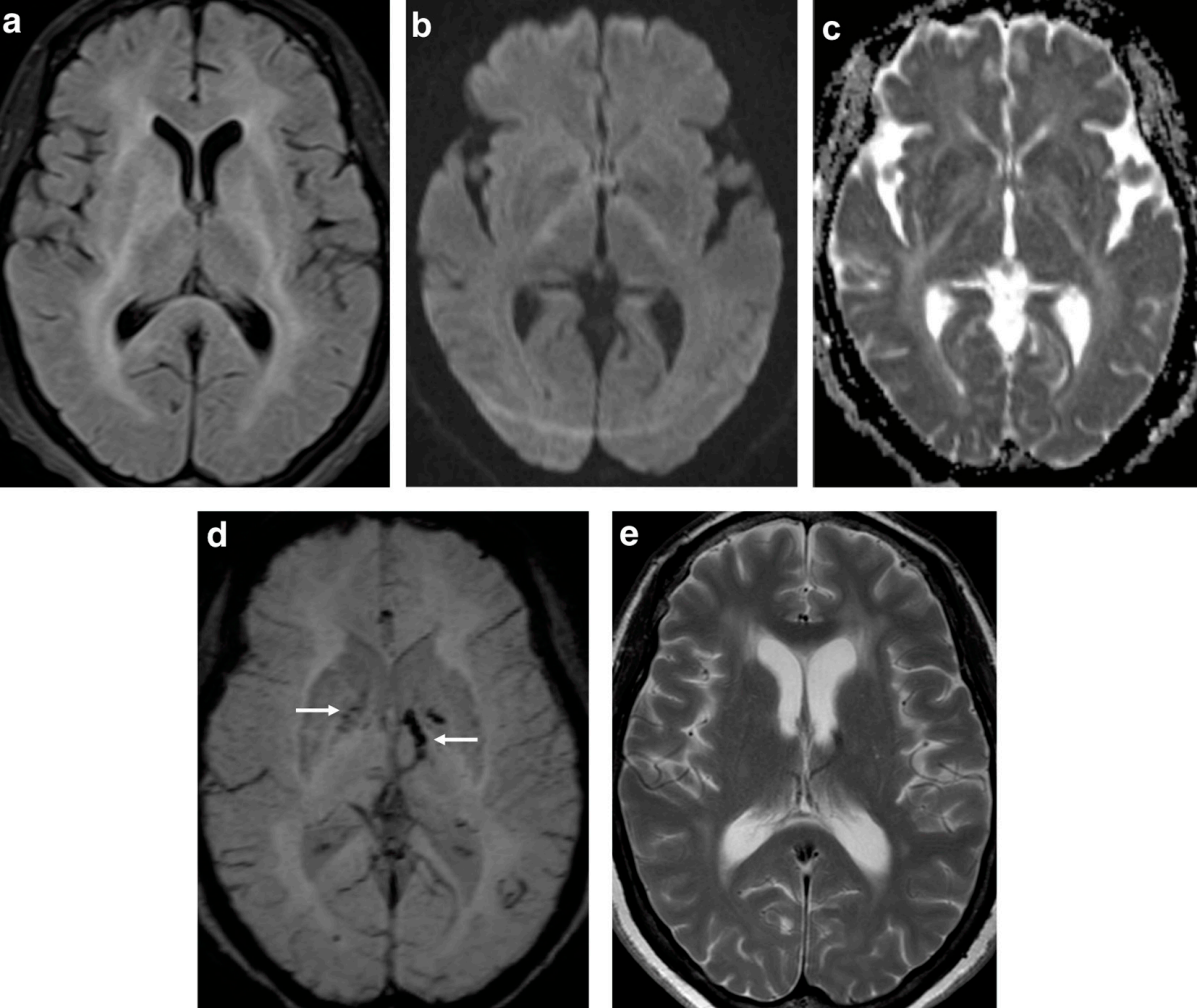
58-year-old female with West African Trypanosomiasis. (a) Axial T2W FLAIR demonstrates bilateral hyperintense signal involving the deep white matter, basal ganglia, internal and external capsule as well as the splenium of the corpus callosum. (b)DWI showing subtle high signal intensity in the posterior limbs of the internal capsules with corresponding low signal on the ADC map consistent with restricted diffusion (c). (d) SWI demonstrating blooming artefact in the globus pallidus bilaterally and left internal capsule suggestive of microhaemorrhages (arrows). (e) Axial *T*
